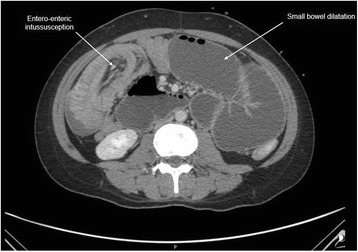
New development of entero-enteric intussusception and small bowel dilatation on subsequent CT
